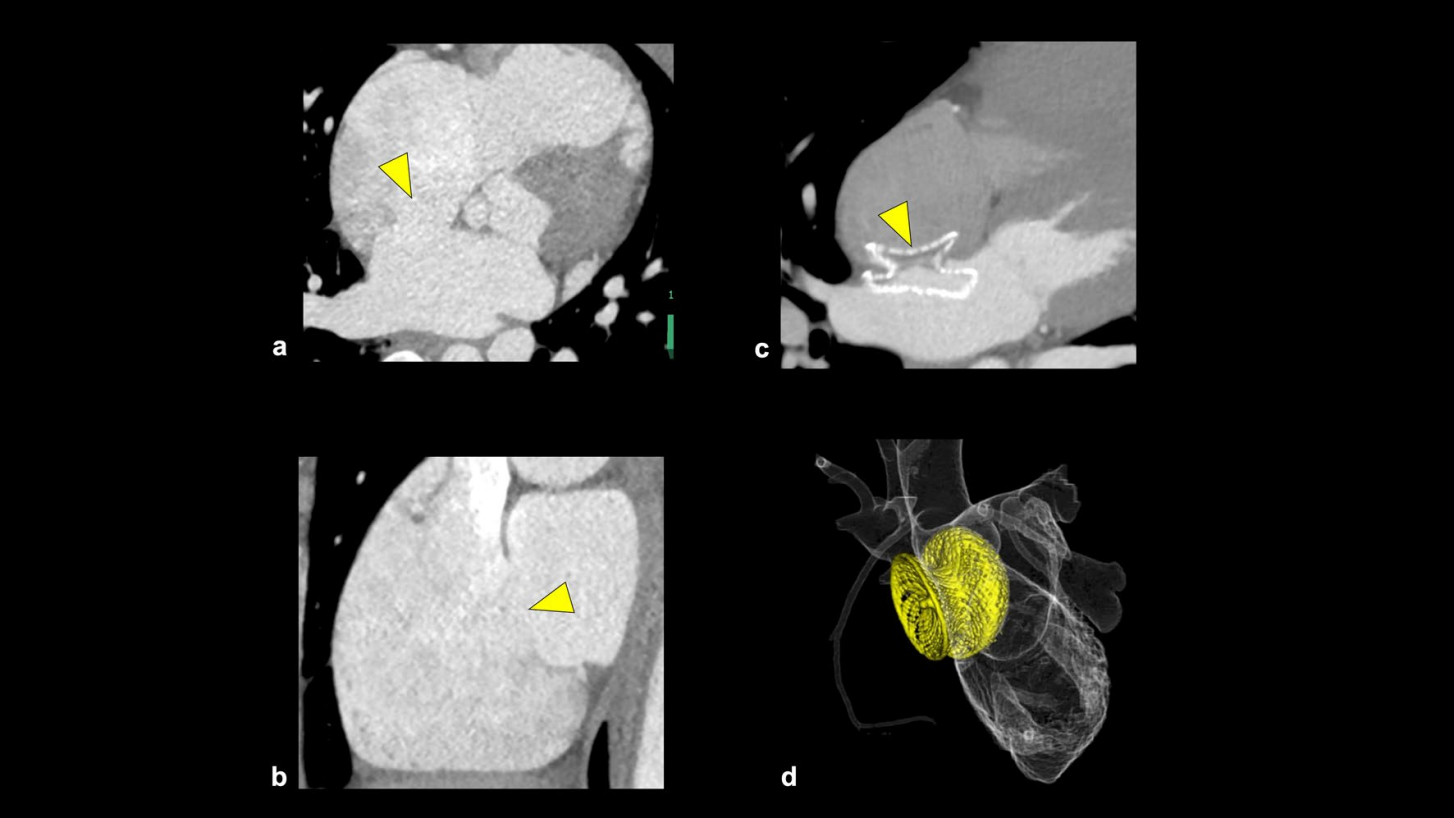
Atrial septal defect. Atrial septal defect (ostium secundum) with an enlarged right atrium is observed on CCTA (a, b: arrowhead). After transcatheter device closure, CCTA allows for assessing atrial septal defect closure (c, d). *CCTA* coronary computed tomography angiography
